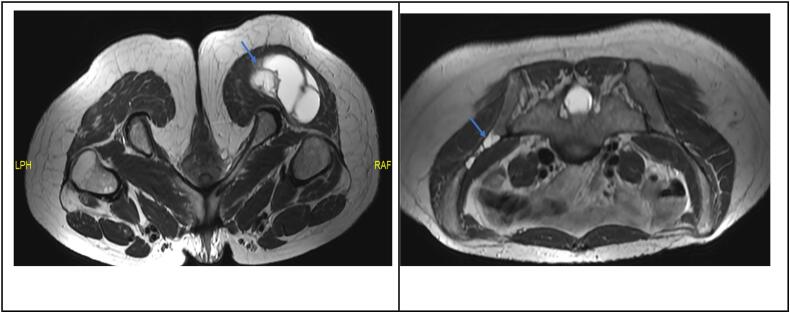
Magnetic resonance images (axial) pointing with blue arrows a well-defined lesion in the right gluteus maximus with a volume of 343 ml, and a left iliac bone lesion consistent with fibrous dysplasia. (For interpretation of the references to colour in this figure legend, the reader is referred to the web version of this article.)
